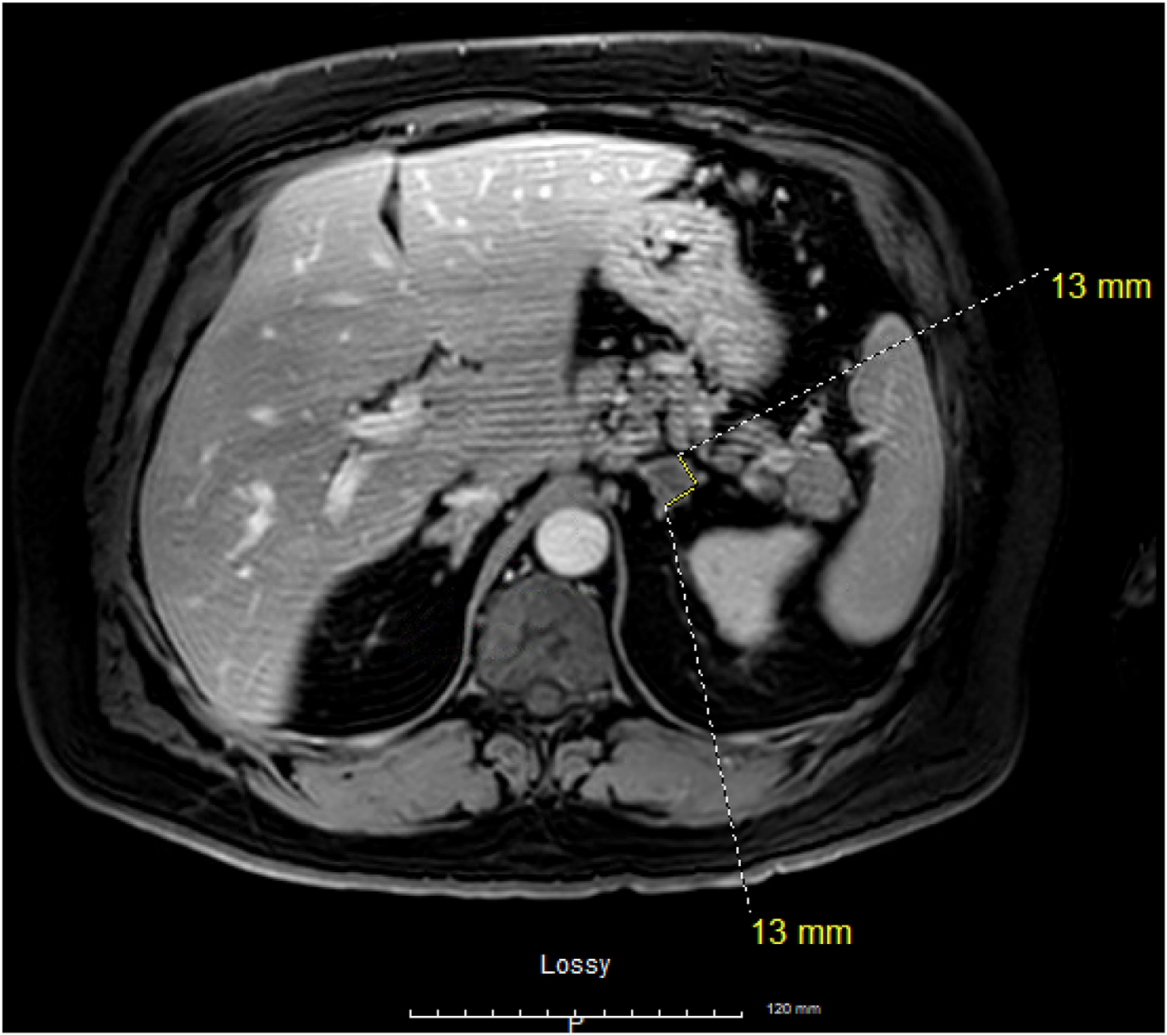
Magnetic resonance imaging (MRI) abdomen during cancer staging showing *left* adrenal tumor of size 13 × 13 mm.
